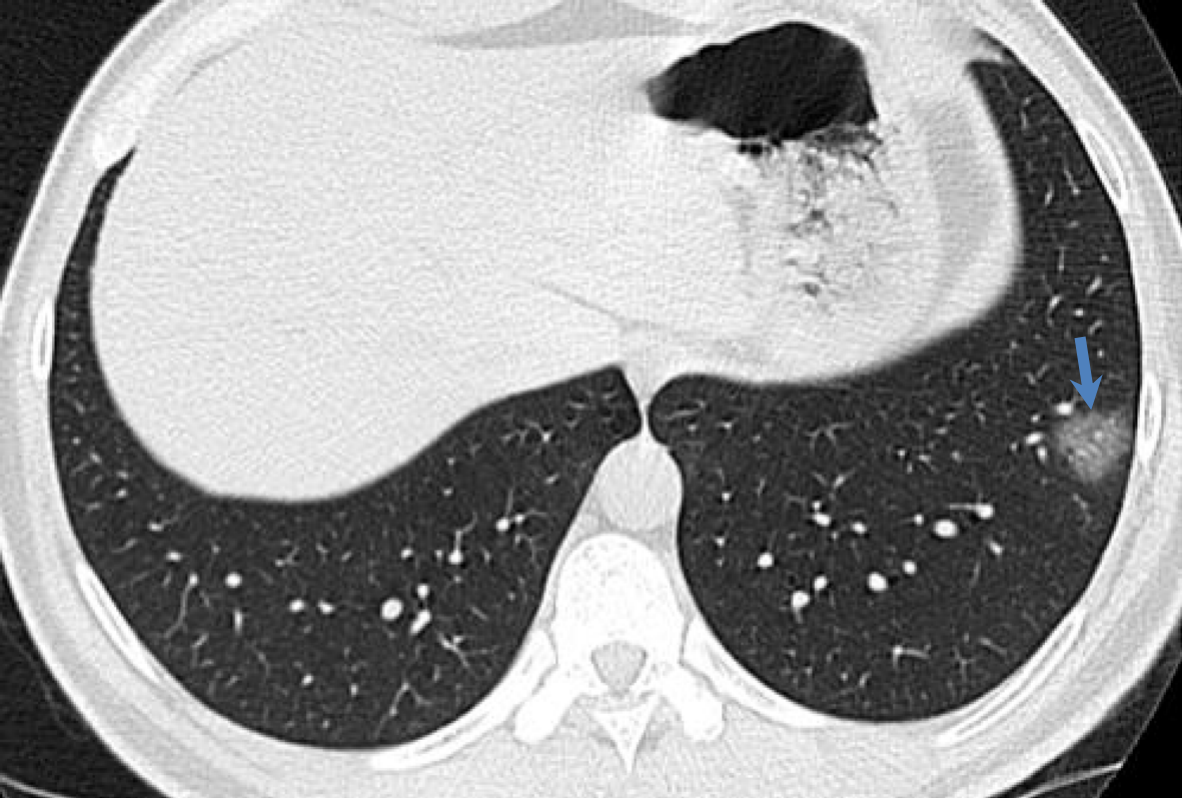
Image of a 36-year-old asymptomatic patient with negative RT-PCR. Pulmonary parenchymal window thin section computed tomography shows ground glass opacity in the left lower lobe of the anterobasal segment of peripheral distribution
